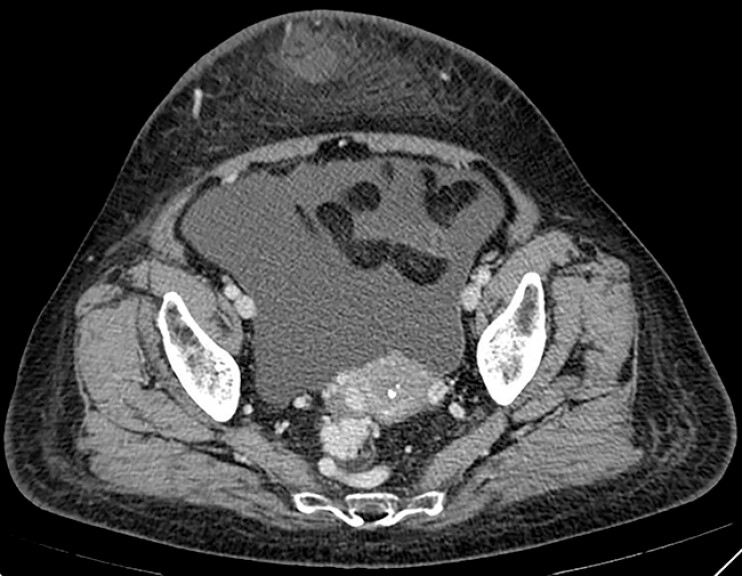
Contrast-enhanced computed tomography image showing the rectal–pelvic variceal plexus and ascites.
